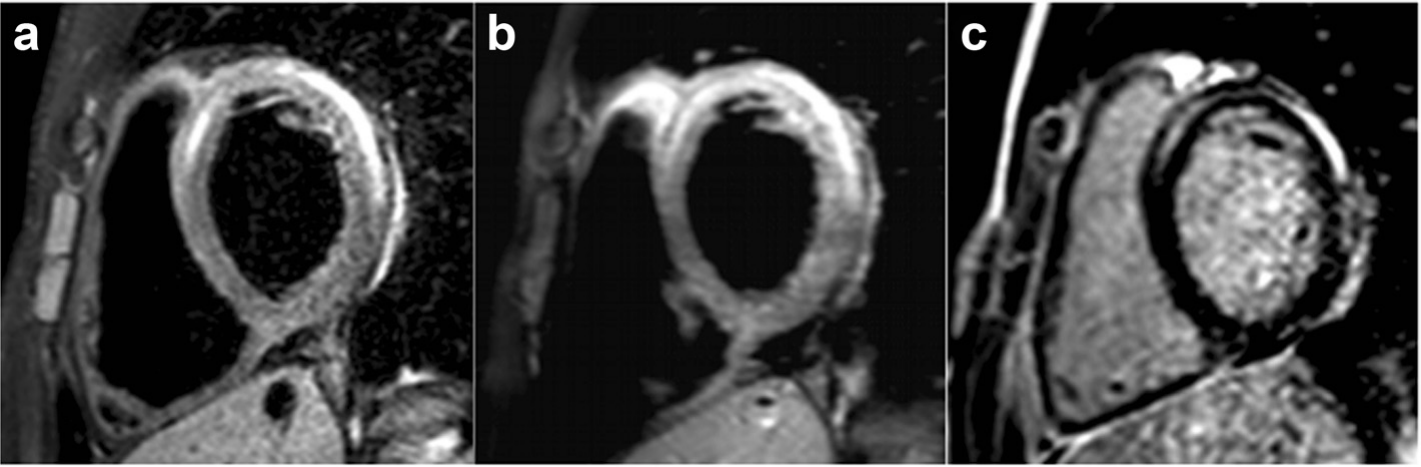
Typical findings of myocarditis on CMR. 16-year-old patient with a midwall and subepicardial distribution of increased signal intensity in the left ventricle on T2-weighted (a), T1-weighted early gadolinium enhancement (b), and late gadolinium enhancement (c) imaging
